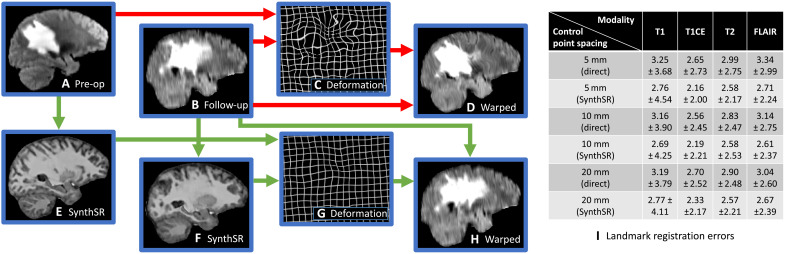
Alignment of preoperative and follow-up brain MRI scans of patients with glioma (BraTS-Reg dataset) using direct registration (red arrows) and SynthSR (green arrows). (A) Sagittal slice of the FLAIR scan of a sample subject (axial acquisition). (B) Approximately corresponding slice of follow-up scan. (C) Deformation field estimated from (A) and (B) with NiftyReg (5-mm control point spacing). (D) Warped follow-up scan. (E and F) SynthSR output of the preoperative and follow-up scans. (G) Deformation field estimated from the synthetic scans. (H) Warped follow-up scan using the deformation field computed with the synthetic images. (I) Mean and SD of landmark error (in millimeters) for different control point spacings and MR contrasts, registering the scans directly versus registering the output of SynthSR. The *P* values for nonparametric Wilcoxon tests comparing the medians are strongly significant in all cases (10^−9^ < *P* < 10^−3^).
